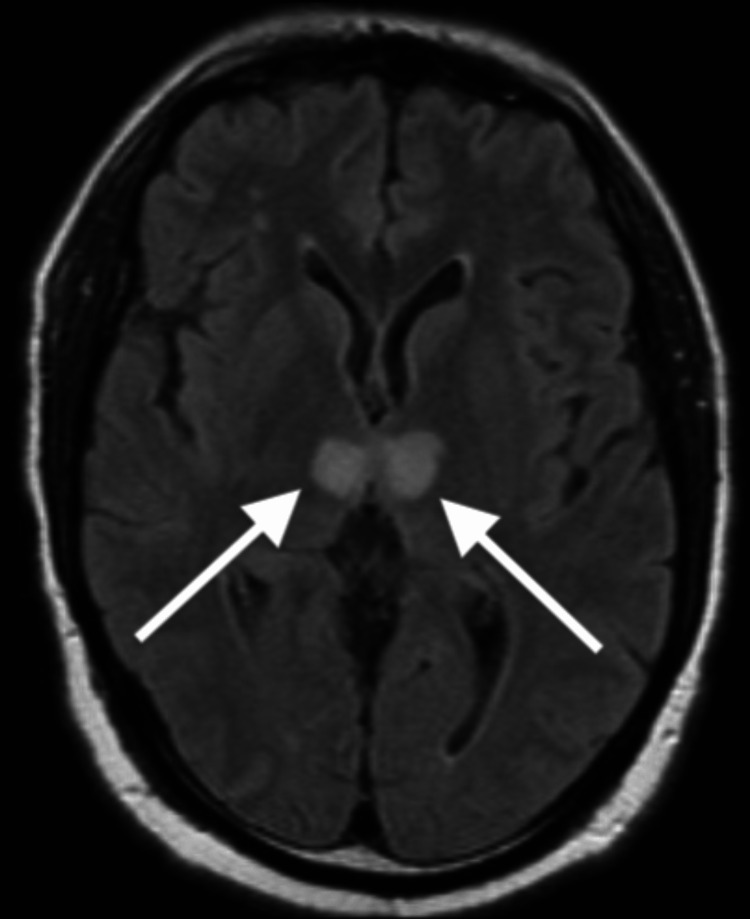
Magnetic resonance imaging of the brain, axial view: T2 fluid-attenuated inversion recovery axial view showing hypoattenuation in both thalami, suggestive of infarcts.
